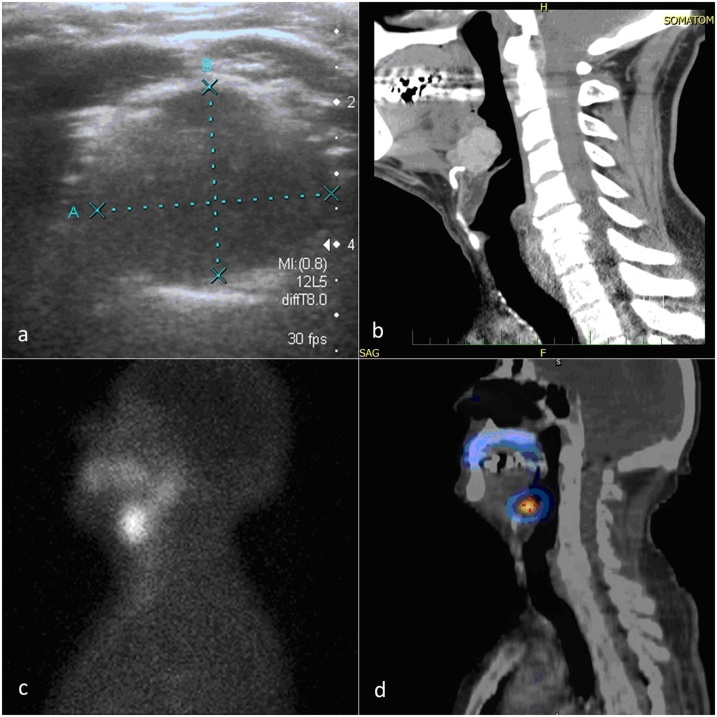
Imaging modalities a) bounded, solid hypoechogenic, slightly vascularised formation 19 × 17 × 9 mm, b) CT scan of lateral projection of heterotopic thyroid tissue in the root of the tongue, without the presence of the thyroid in the usual neck position, c) scintigraphy and, d) SPECT (single photon emission computed tomography) radiopharmaceuticals (^99m^Tc-pertechnetate) in the root of the tongue and confirmed the absence of thyroid tissue in its usual location in the anterior neck.
